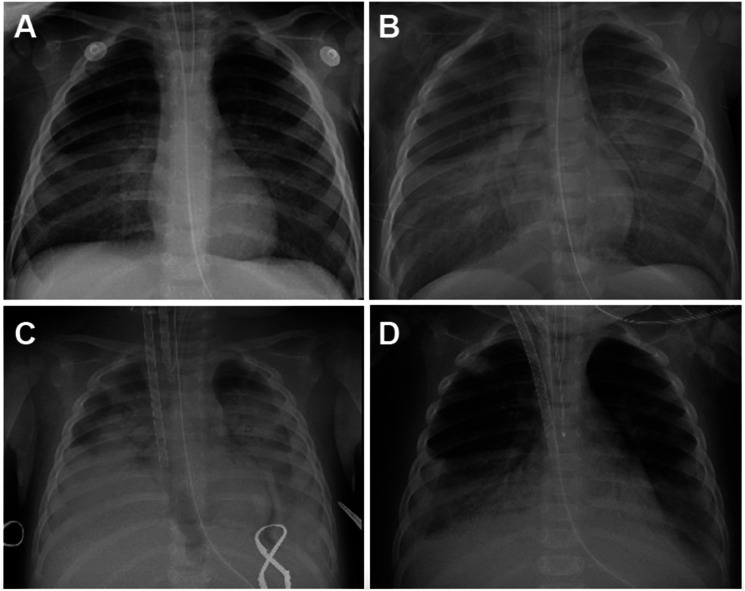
Progression of chest radiographs through intubation and ECMO cannulation.
